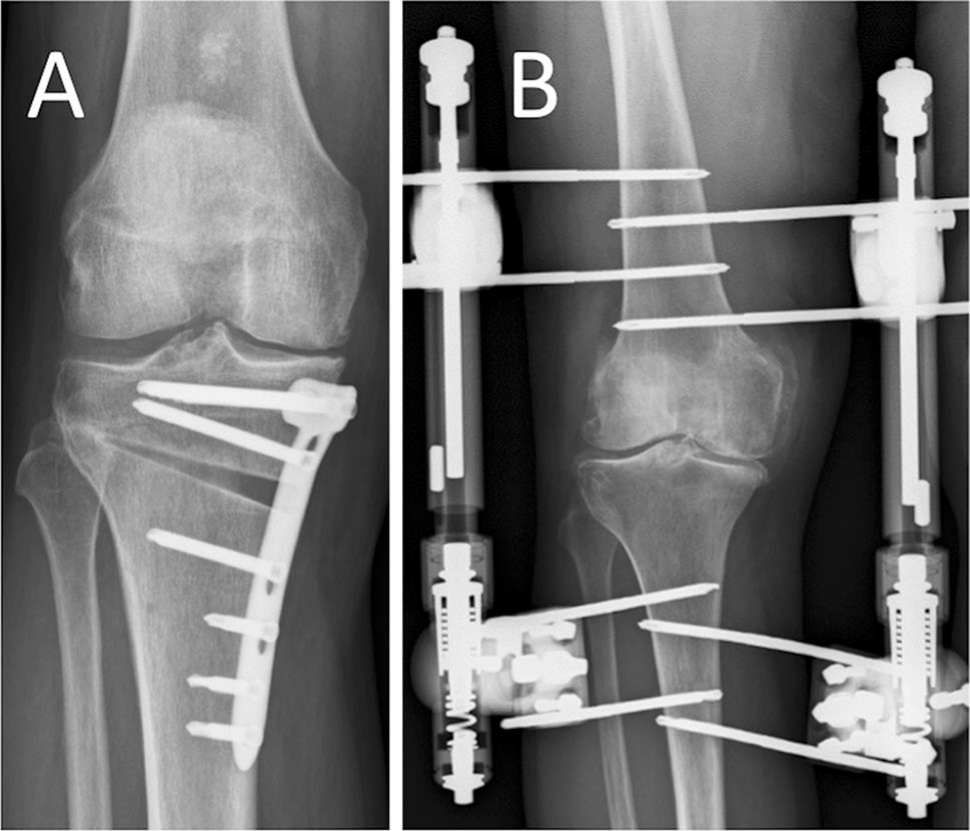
Example of a post-operative radiograph, a patient treated with HTO, b patient treated with knee joint distraction
